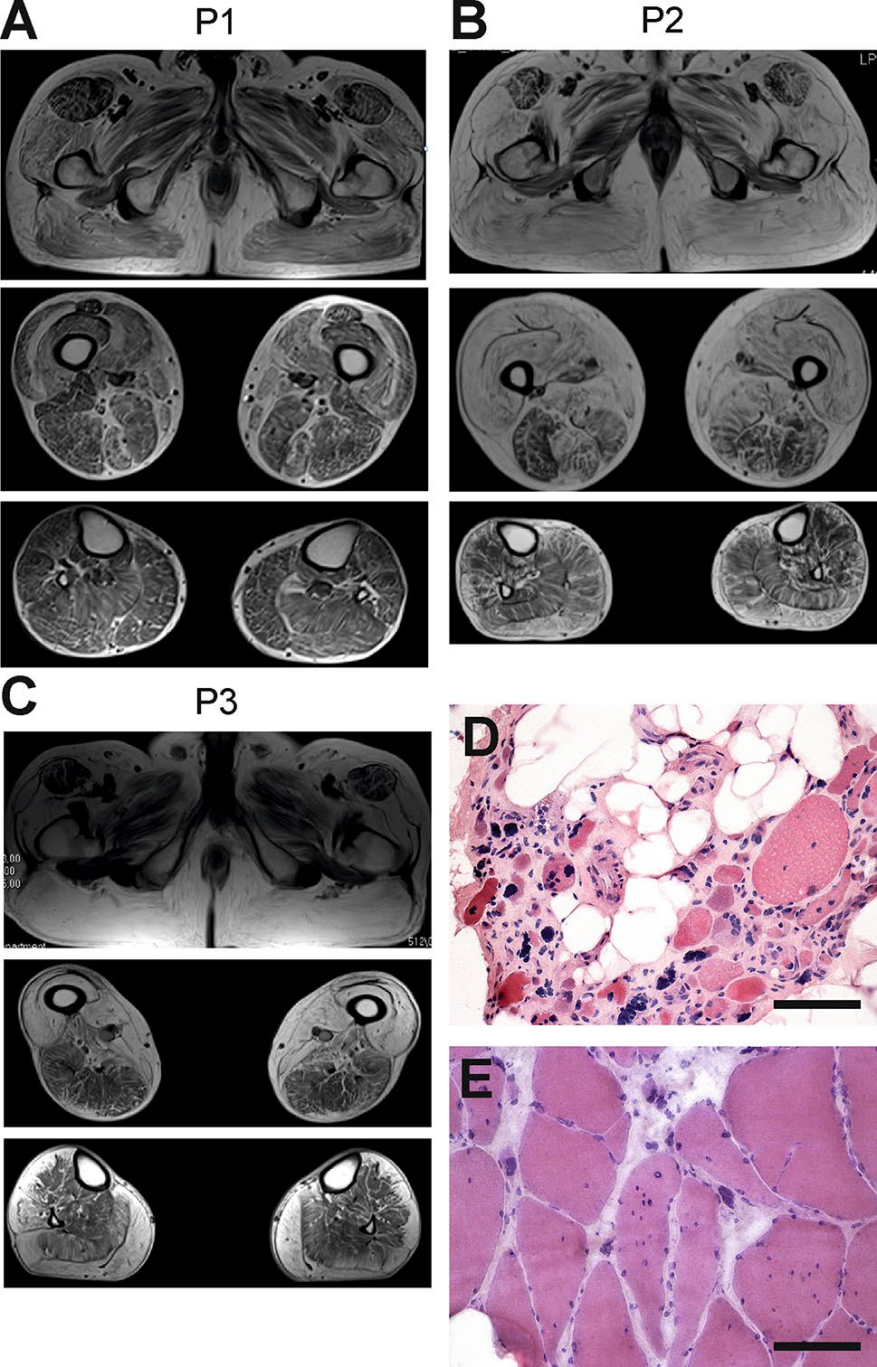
Muscle MRI and biopsies. MRI was performed for a individual P1 at age 60, b individual P2 at age 59, and c individual P3 at age 65. Gluteus maximus, quadriceps, sartorius and gracilis muscles were most severely affected in all patients, although the most proximal parts of the rectus femoris muscles were relatively spared or even hypertrophic. Anterior thigh muscles were always more severely affected than the hamstrings and adductor compartments. A very peculiar pattern of fatty degeneration was observed in patients P2 and P3, where only the outer parts of the anterior and lateral compartment muscles were replaced by fat. In patient P1, there was an unusual crescent-shaped fatty-degeneration involving the inner parts of vastus lateralis and medialis muscles. Muscle biopsy (vastus lateralis) from d individual P2 and e individual P1 showed a dystrophic pattern, where individual P2 had an almost end-stage pathology with severe atrophy, fibrosis and fatty infiltration. Scale bars 100µm
